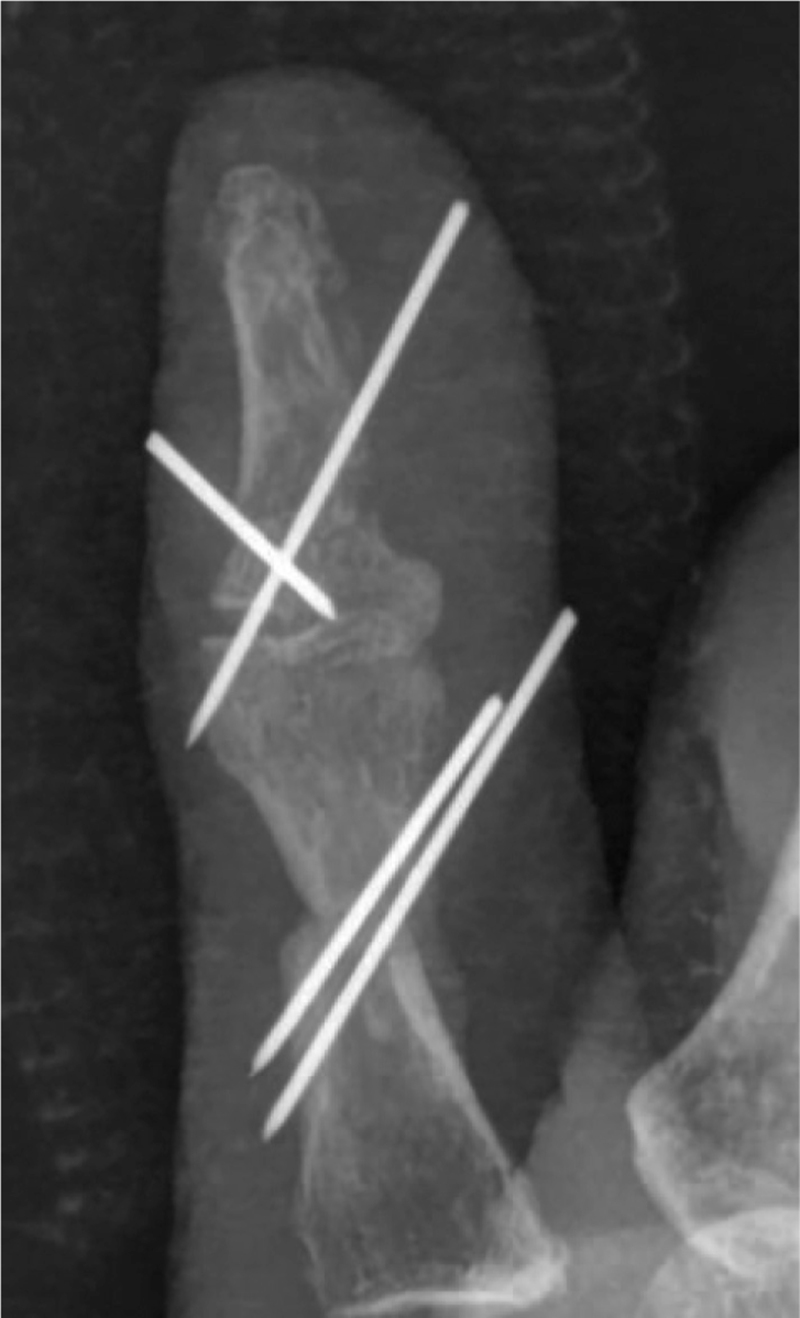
Orthopantomogram of the affected finger 2 months after surgery.
